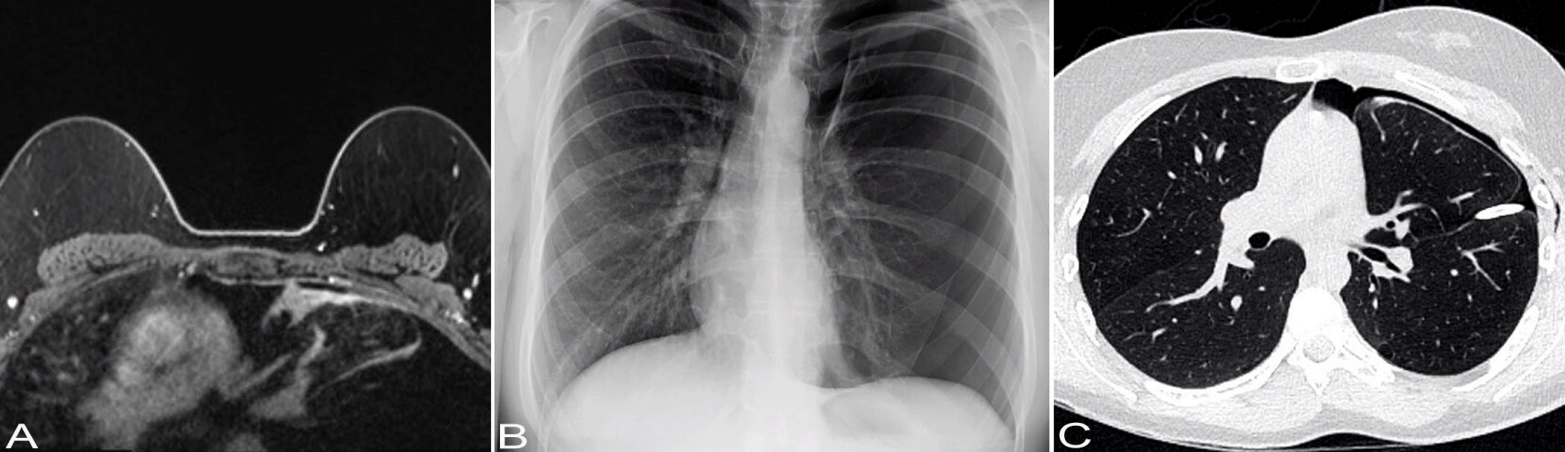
Imaging findings. (A) Axial non-contrast T1-weighted breast MRI image shows no evidence of malignancy. There is a mediastinal shift with the heart shifted to the right and a lack of lung markings in the upper left lung, suggesting the possibility of bulla in the upper left lung. (B) Chest X-ray shows a left-sided pneumothorax with cardiac and mediastinal shift rightward suggesting underlying tension component. (C) Chest CT shows a small left anterior pneumothorax. There is a left-sided pleural drainage catheter with its tip along the left major fissure. There are subtle patchy consolidative opacities in the subpleural aspects of the left upper lobe.
